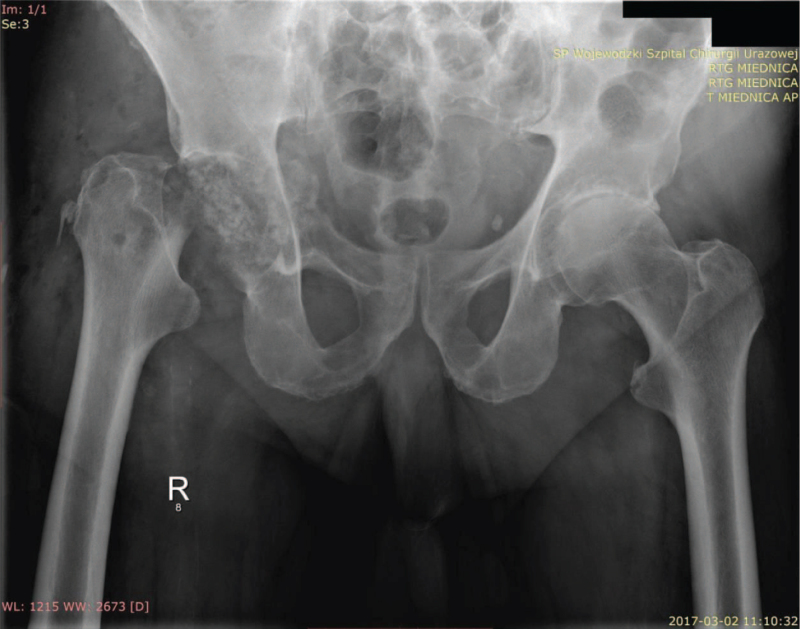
X-ray examination of the pelvis with hip joints. Condition after removal of the ASR implant, leaving the so-called “hanging joint” with visible extensive plastic surgery with allogeneic cancellous bone of the bottom of the acetabulum of the right hip joint, degenerative changes in the left hip.
